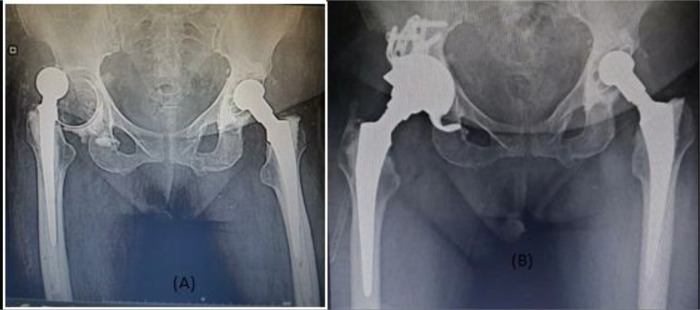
(A) Preoperative X-ray for a case with acetabular aseptic loosening, (B) postoperative X-ray after revision using Delta TT revision system with stem retention.
